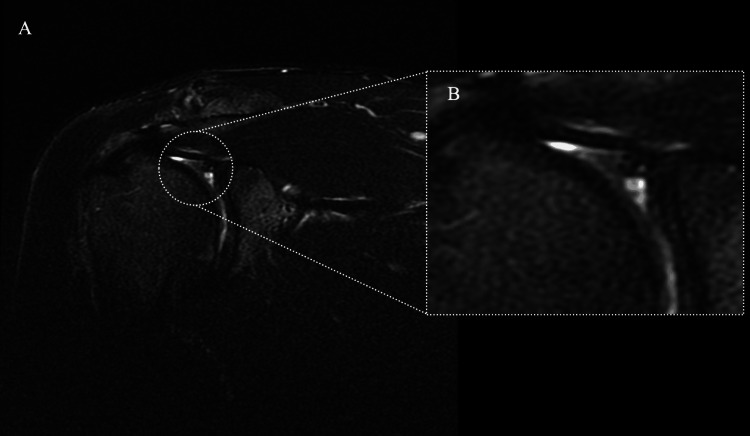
Preoperative T2 fat-suppressed magnetic resonance imaging (MRI) of the right shoulder. Preoperative MRI showing a type I superior labrum anterior to posterior (SLAP) lesion. (A) Coronal view of the right shoulder; (B) Magnified view of the lesion in the superior labrum.
